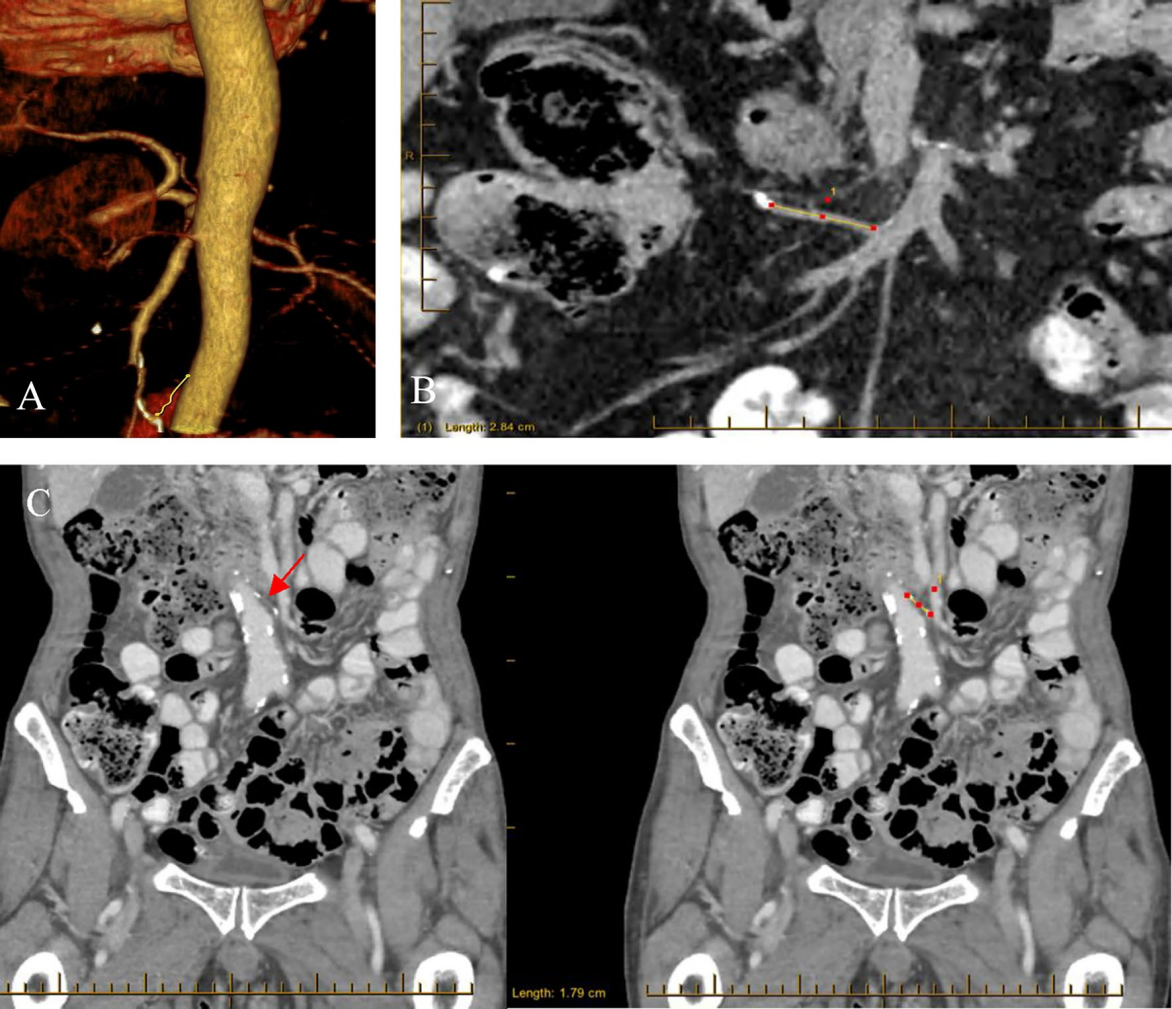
A Coronal volume-rendered reformatted CT image of the IMA RAPL, defined by its termination at a surgical clip; B Multi-planar reconstructed (MPR) CT image with ICA RAPL defined and measured in the coronal plane; C MPR CT image with IMA RAPL defined (red arrow) and measured in the coronal plane. Surgical clip/ in (B) and granuloma in (C) are denoted by hyper-density at maximal limits of the measurement
